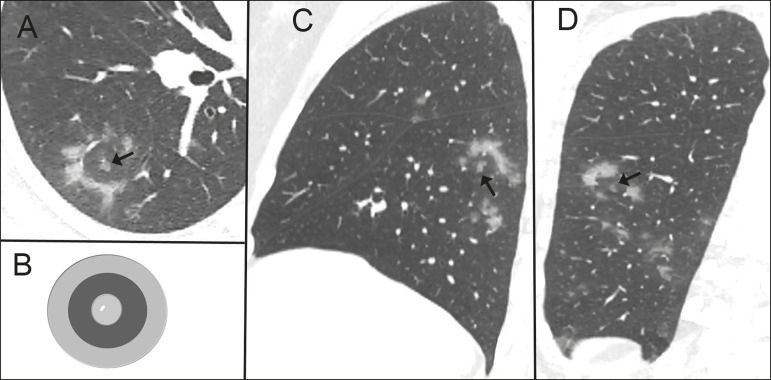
Nonenhanced cross-sectional CT image of the right lung (A) in a 37-year-old woman with COVID-19 pneumonia and minimal dyspnea shows an irregular ring-like opacity containing in its center a small nodular ground-glass opacity surrounding a very small vessel (arrow). The combination of central nodular opacity and the peripheral ring of increased opacity resemble a shooting target (B) thus resulting in a CT target sign. The target sign can also be seen in the sagittal (C, arrow) and coronal (D, arrow) reformations. Also noted are small patchy ground-glass opacities.
